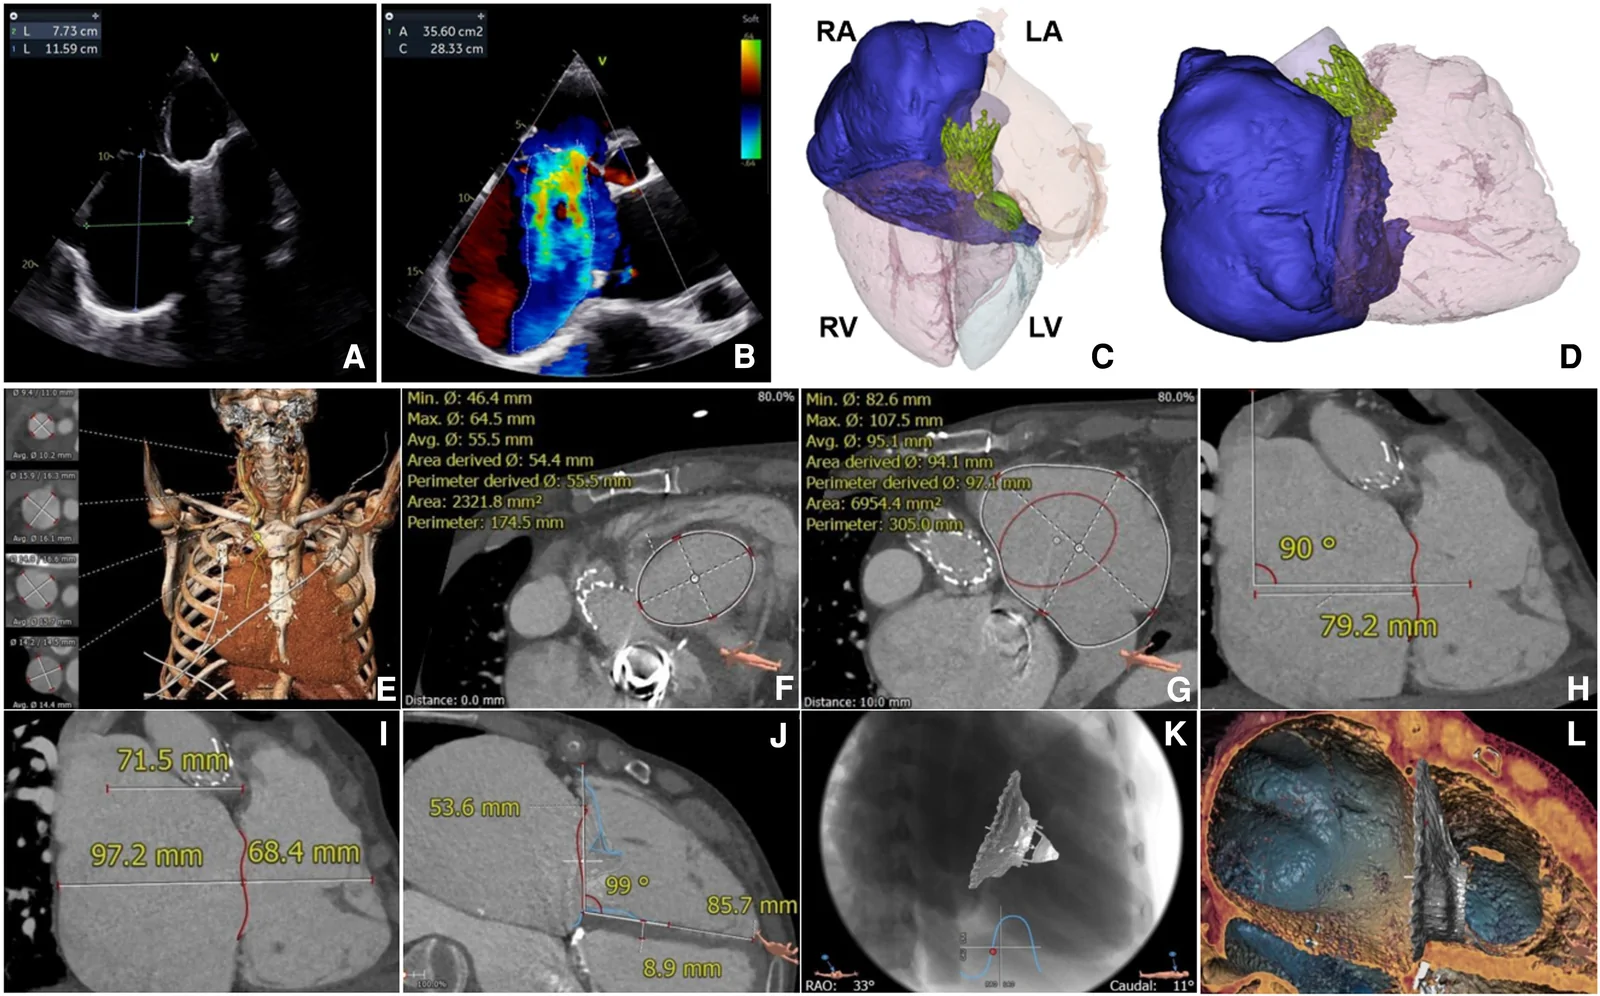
Preprocedural multimodality imaging and CTA-based procedural planning. (*A*, *B*) TTE showed marked right atrial enlargement (11.59 × 7.73 cm) and torrential tricuspid regurgitation with a colour Doppler jet area of 35.6 cm^2^. (*C*, *D*) 3D CTA reconstruction demonstrated a giant right atrium and distorted right-heart geometry. (*E–G*) CTA-based planning assessed the transjugular access route, annular dimensions, and dimensions 10 mm above the annular plane. (*H–L*) Additional measurements included the planned bend-point-to-annulus distance (79.2 mm), right ventricular working length, annulus–septal angle, septal thickness, and fluoroscopic deployment projection.
